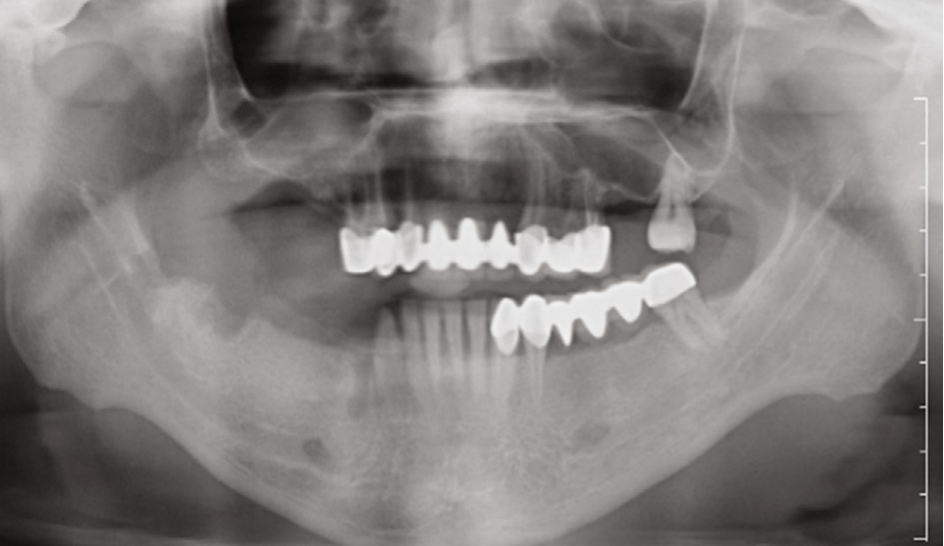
Postoperative orthopantomograph of the patient.
